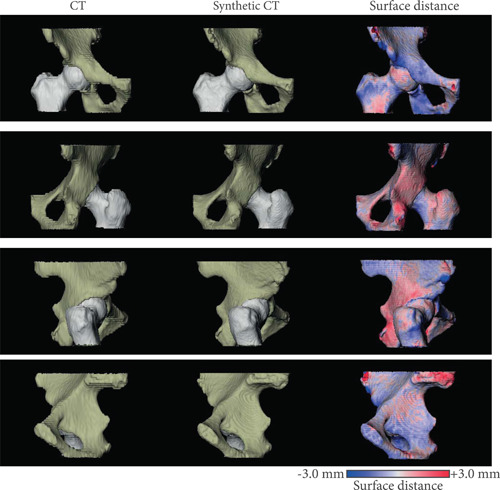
Bone models obtained for a right femur and pelvis as seen from four different views (from top to bottom: anterior, posterior, right, and left views). The corresponding sCT‐to‐CT surface distances are mapped on the sCT bone model. Negative values indicate the sCT model is larger. CT, computed tomography; sCT, synthetic computed tomography [Color figure can be viewed at wileyonlinelibrary.com]
